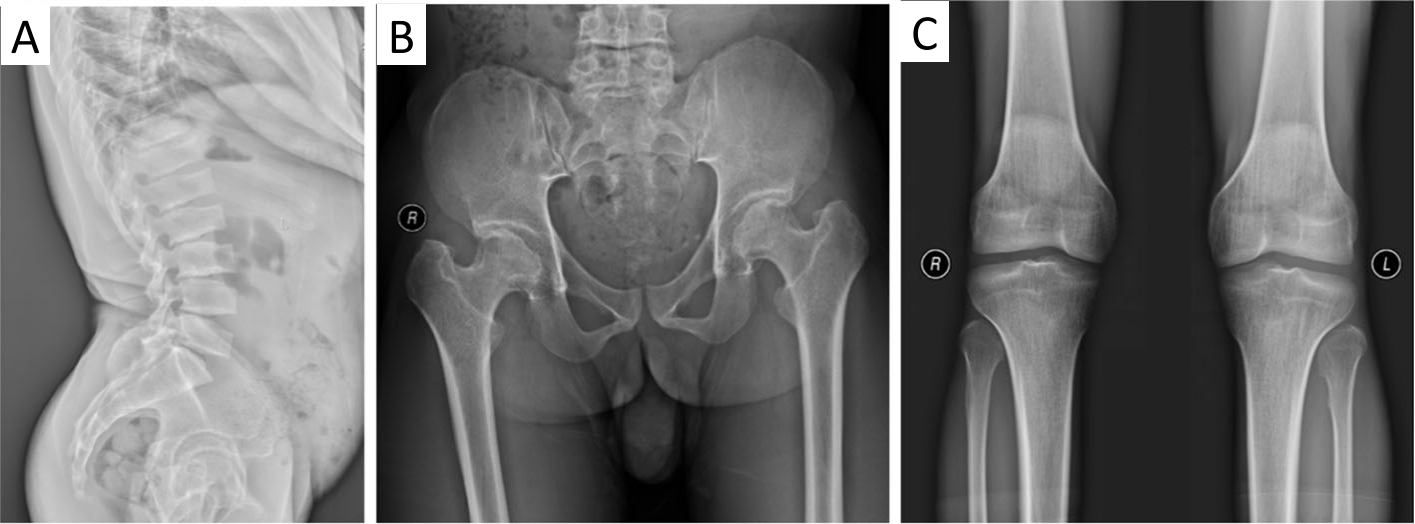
X-ray radiographs of the proband. a, the radiograph of the lateral lumbosacral spine showed platyspondyly and dysplasia with posterior humping. b, the radiograph of the hips showed flattened femoral heads, narrow hip-joint surfaces, and osteosclerosis of the pelvis. c, the radiograph of the knees displayed flattened tibial plateaus and irregular surface of lateral femur condyles
